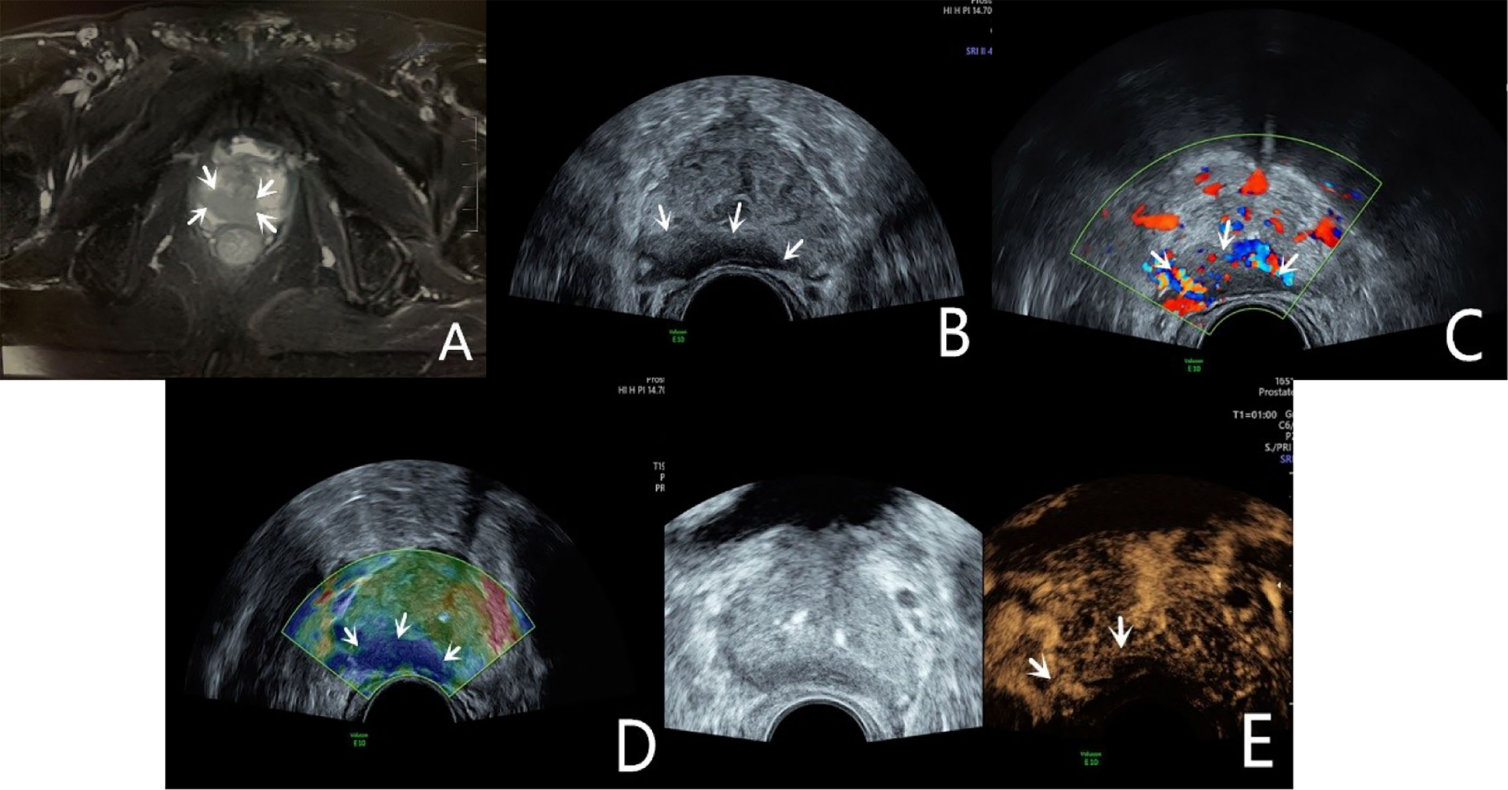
Diagnosis of PCa using mpUS—case 1. A 68-year-old patient has a total serum PSA of 10.4ng/ml. The T2-weighted sequence of MRI (A, arrow) shows a slightly low signal shadow in the peripheral zone of the prostate, suggesting PCa in the diagnosis. The lesion showed a slightly hypoechoic area on the B-mode (B, arrow), and it’s not clearly demarcated from the seminal vesicle gland. CDU shows an abnormally increased blood flow in the lesion (C, arrow). TRTE shows that the slightly hypoechoic area of the prostate’s peripheral zone is highly stiff (D, arrow). CEUS shows early high enhancement within the lesion (E, arrow). TRUS-guided systematic biopsy confirmed that the peripheral zone of the prostate was a Gleason 4 + 4 PCa.
